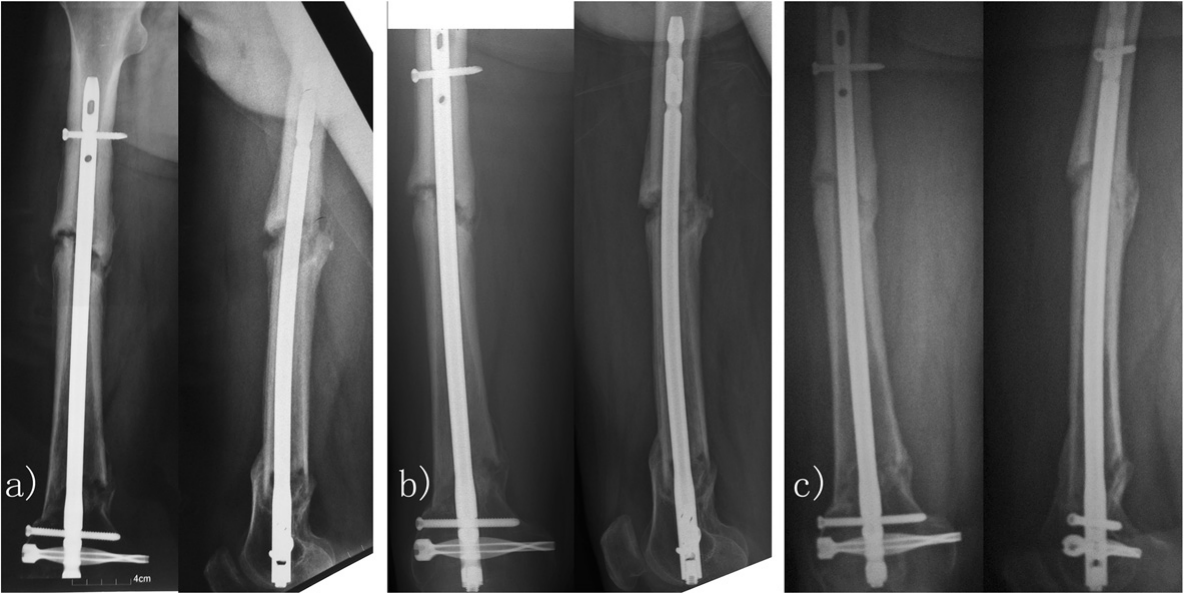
Delayed union of femoral fracture treated with PEMF. (a) PEMF treatment was started in a 59-year-old male patient who received reduction and intramedullary fixation 5 months ago; (b) Radiographies showed progress to union following 3 months of treatment; (c) Fracture united after 8 months of treatment.
